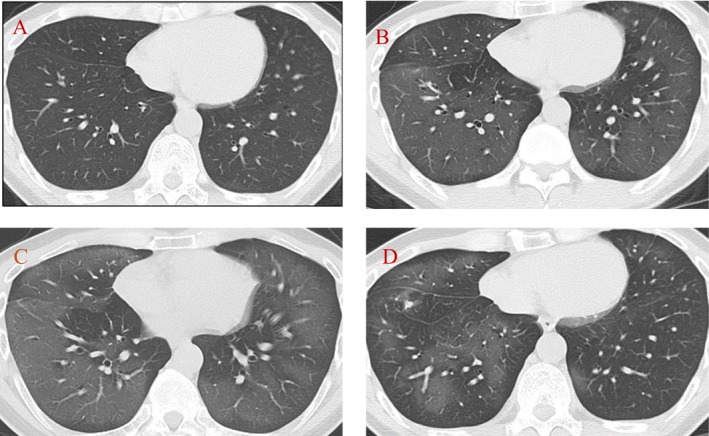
Progression of computed tomography findings. (A) During the first visit to our hospital, no abnormal findings were observed in either lung. (B) During the first hospitalization in December 2020, ground‐glass opacities appeared with areas of geographic sparing in both lungs. (C) During the second hospitalization in January 2021, ground‐glass opacities remained in both lungs. (D) In February 2021, ground‐glass opacities began resolving in both lungs.
